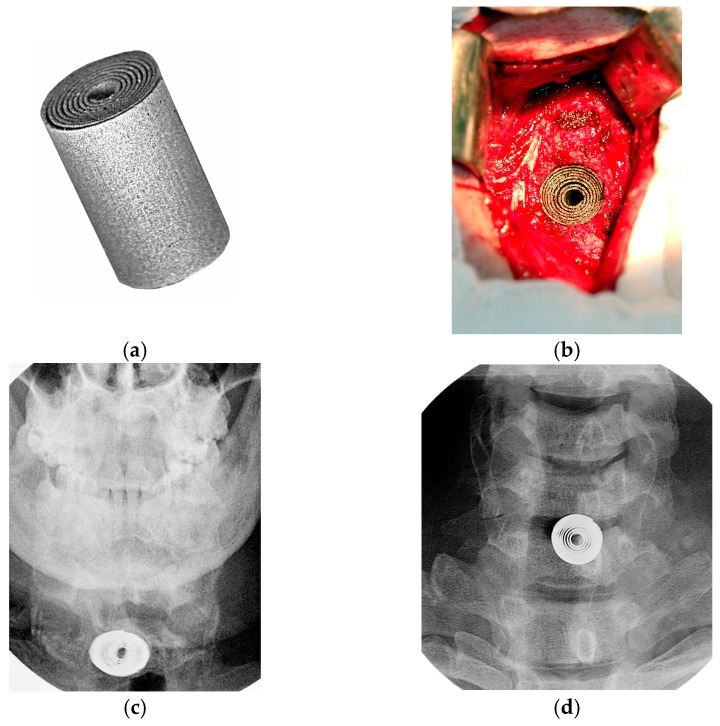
Cervical spine dynamic PTN cage: (a) General view; (b) intraoperative view of the surgical wound; X-ray images of the head tilted (c) down and (d) back in two years after surgery [101].
